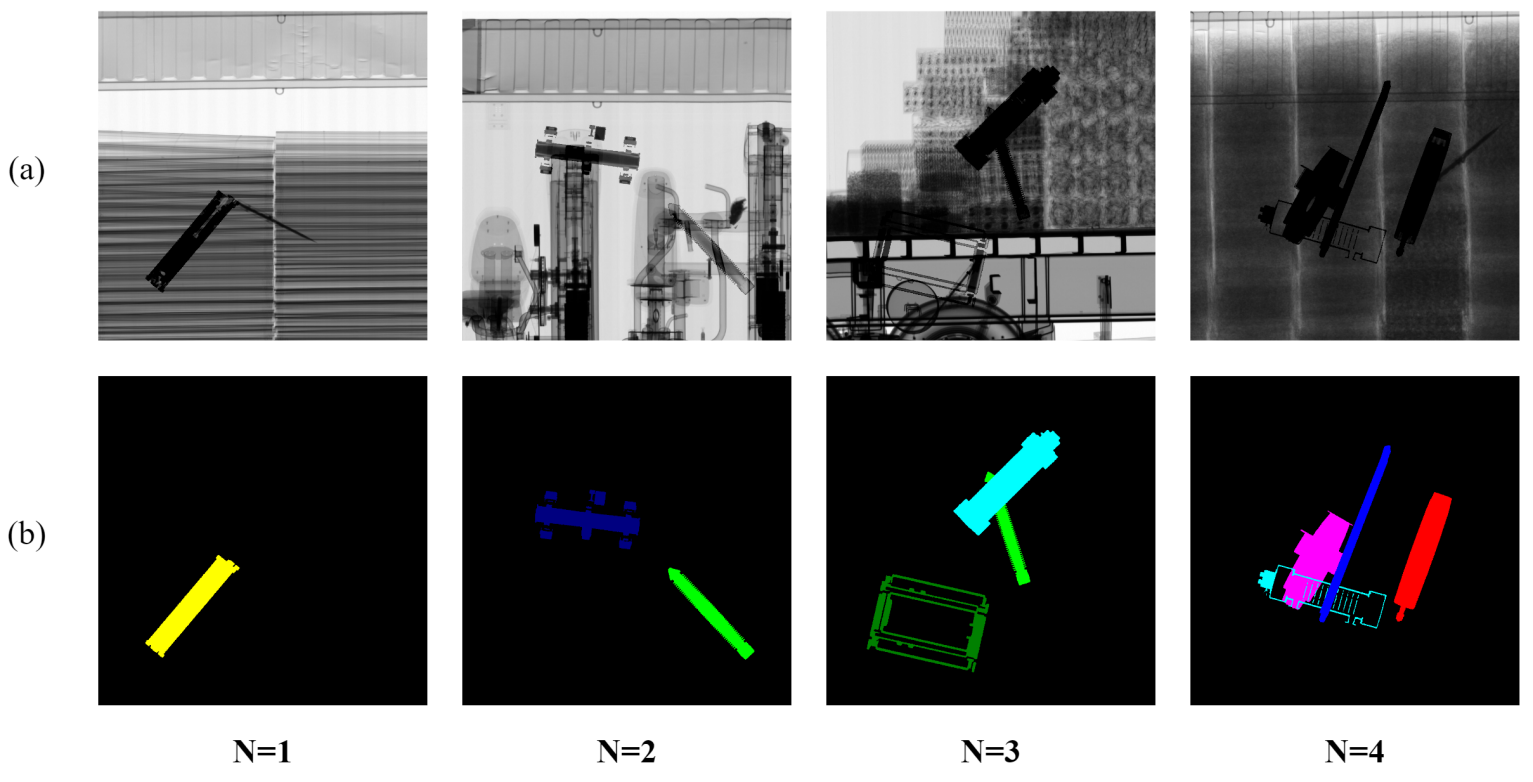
NuclearCargoX examples with changes in the number of nuclear items (N): (a) synthetic X-ray images generated using the proposed method and (b) corresponding labels for the synthetic X-ray images.
